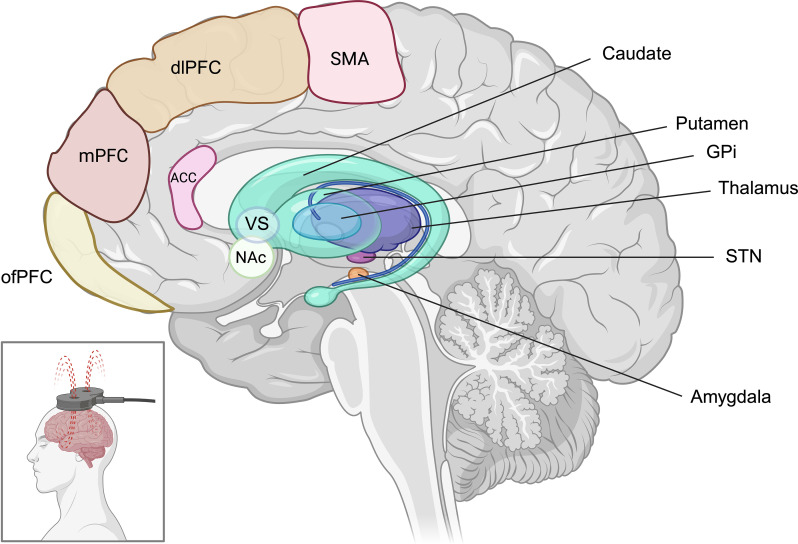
Deep targets accessible by neurosurgical lesions, deep brain stimulation (DBS), and focused ultrasound (FUS).
